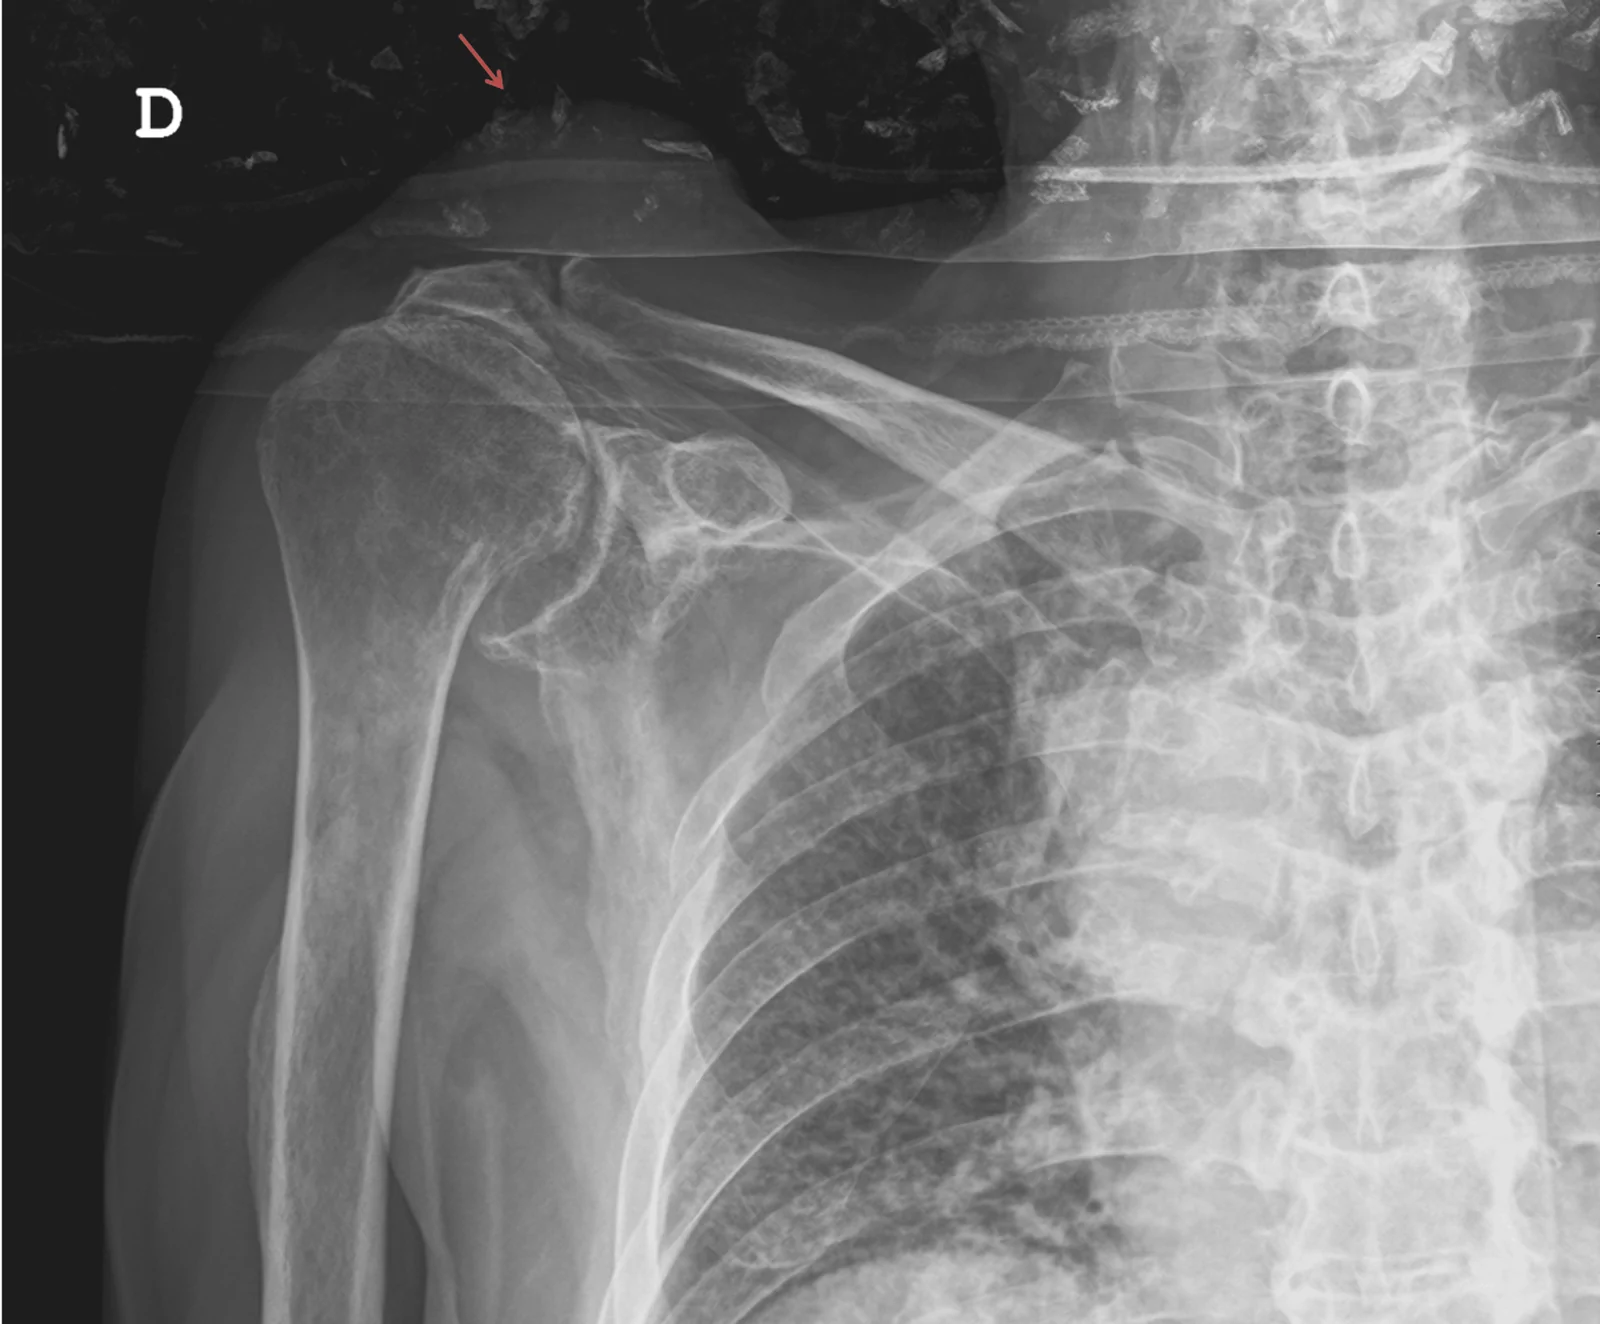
Right shoulder radiograph showing soft-tissue swelling superior to the ACJ (arrow), advanced degenerative changes of the GHJ and ACJ, superior migration of the humeral head, and narrowing of the subacromial space. ACJ, acromioclavicular joint; GHJ, glenohumeral joint
